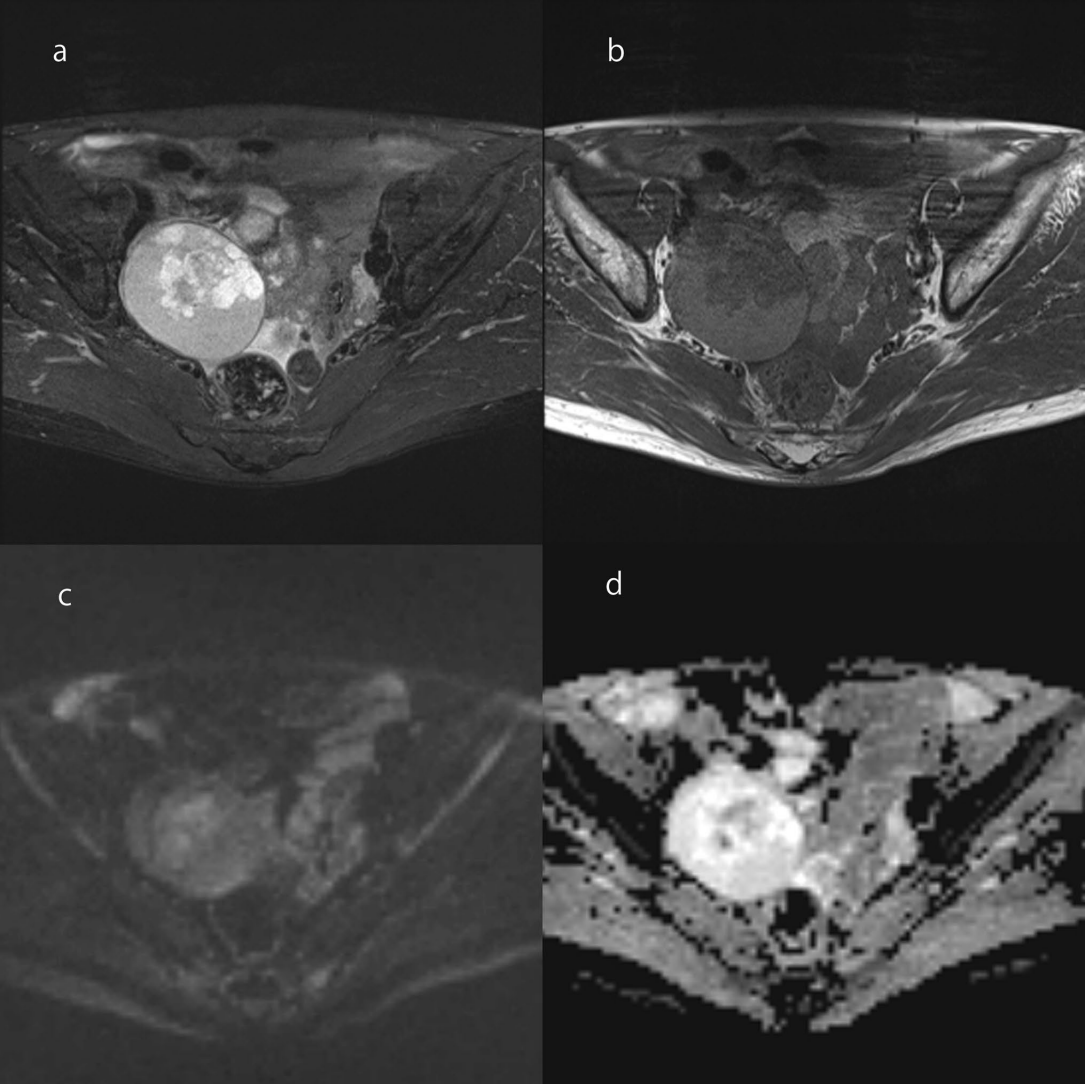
Seromucinous borderline tumor in a woman in her 60 s. a FS-T2WI shows the right ovarian cystic mass to have slightly hyperintense content with a papillary mural nodule. The papillary mural nodule shows marked hyperintensity with central dendritic hypo-intensities. This finding resembles those of serous borderline tumor. b On T1WI, the right ovarian cystic mass has slightly hyperintense content with a hypointense mural nodule. c On DWI (high b value), the central area of the mural nodule is slightly hyperintense. d On ADC maps, the central area of the mural nodule is slightly hypointense but the peripheral area of the mural nodule is markedly hyperintense
